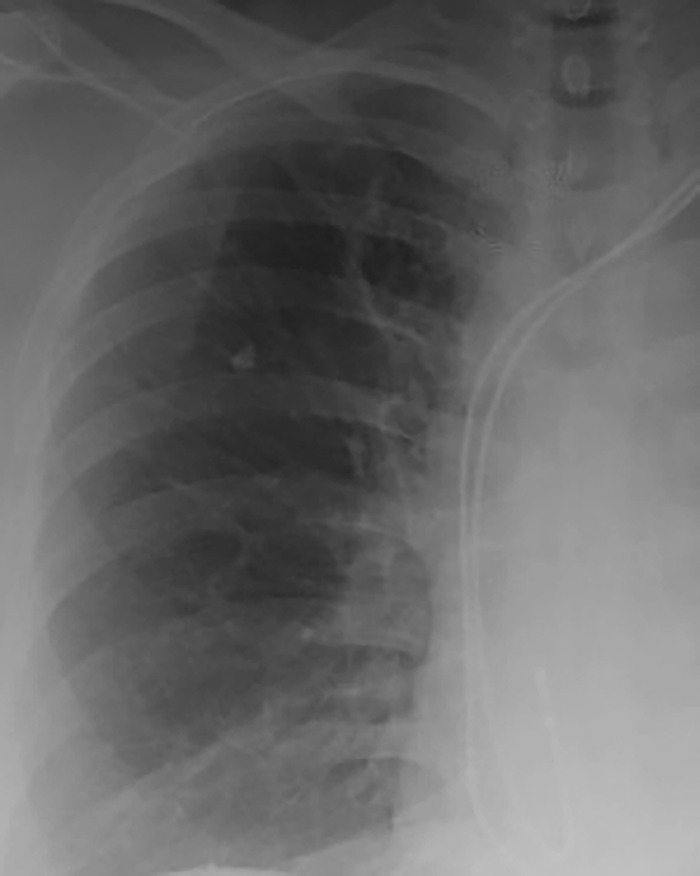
Postoperative chest x-ray shows resolution of the paratracheal mass.
